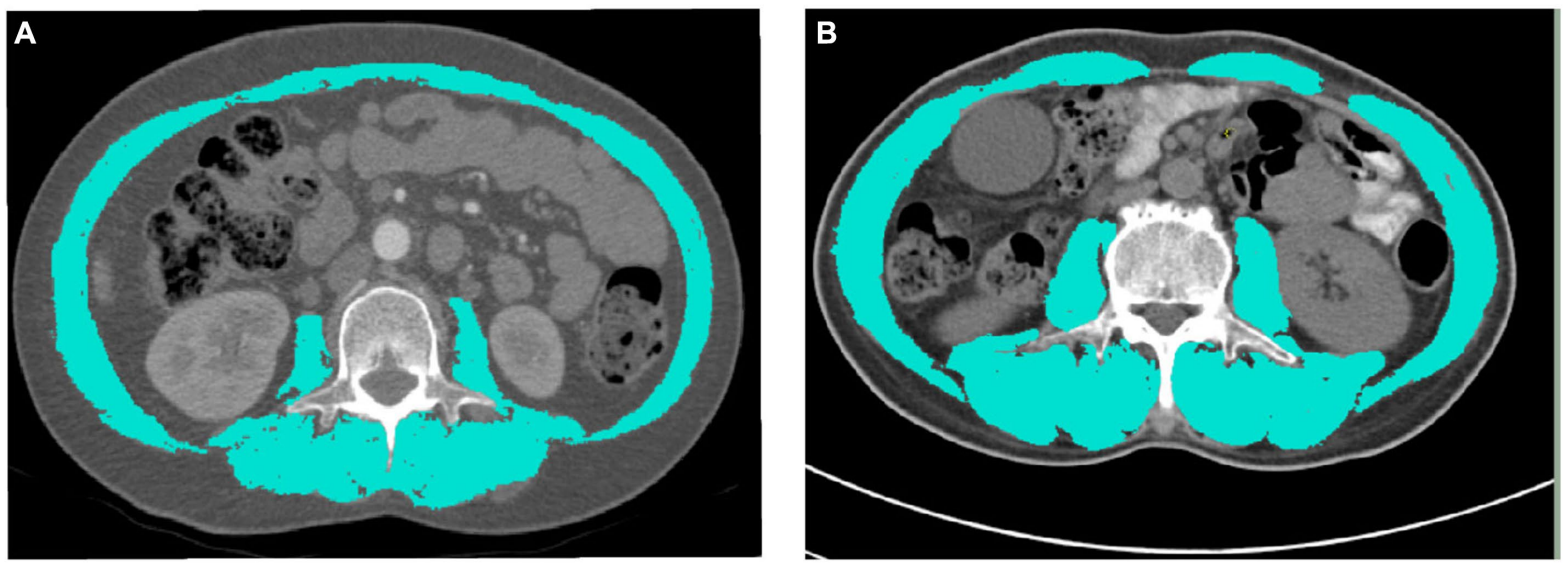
The skeletal muscle index (SMI) of the third lumbar vertebra on CT images. (A) The SMI was 34.73cm^2^/m^2^ in a female patient. (B) A male patient with a SMI of 48.20cm^2^/m^2^.
